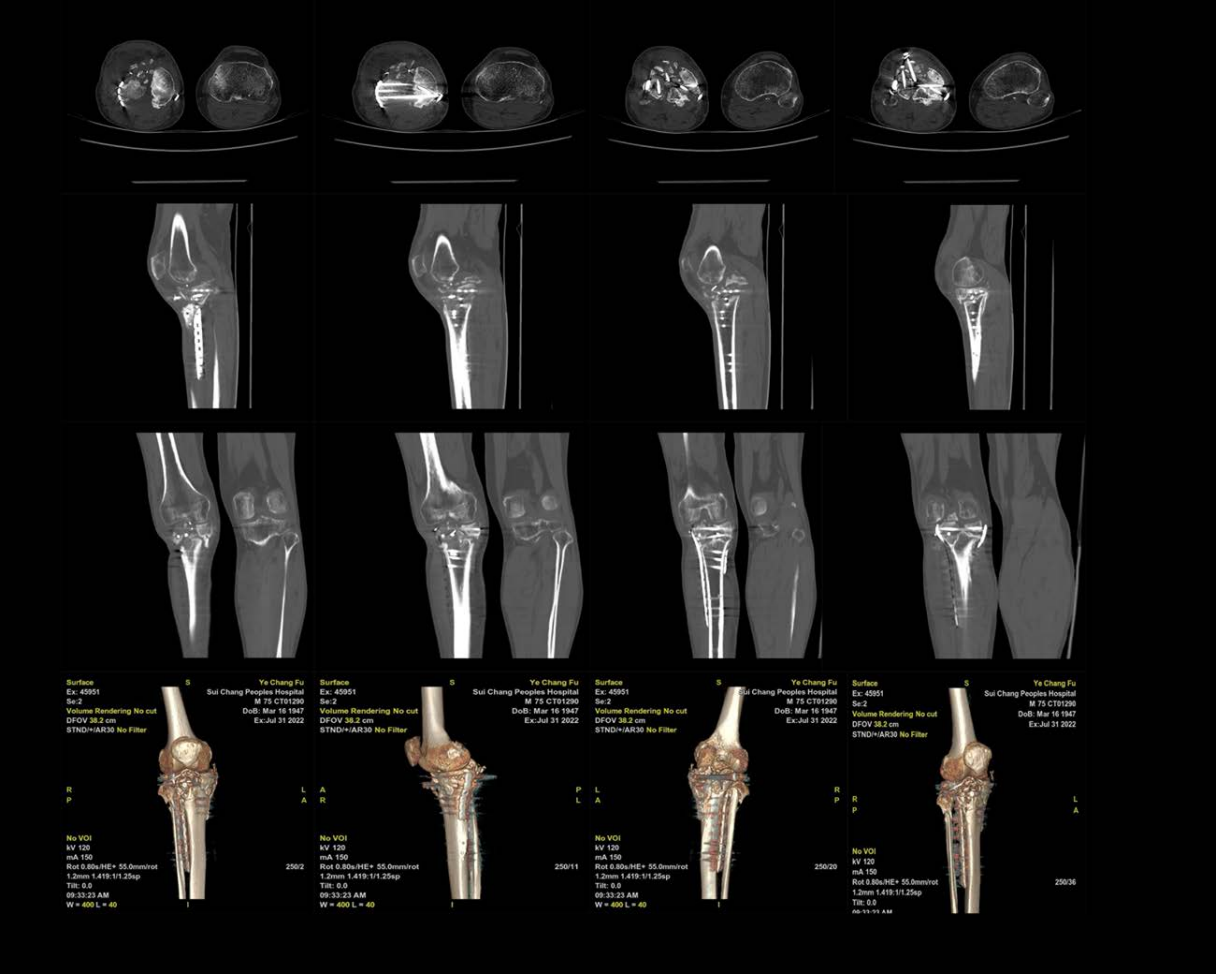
Computed tomography revealed delayed fracture healing, with some deformities approximately 12 months after surgery (Fig. 6; date of examination: July 31, 2022). Axial (A; B; C; D), agittal (E; F; G; H), coronal (I; J; K; L), and 3-dimensional (M; N; O; P) CT.
